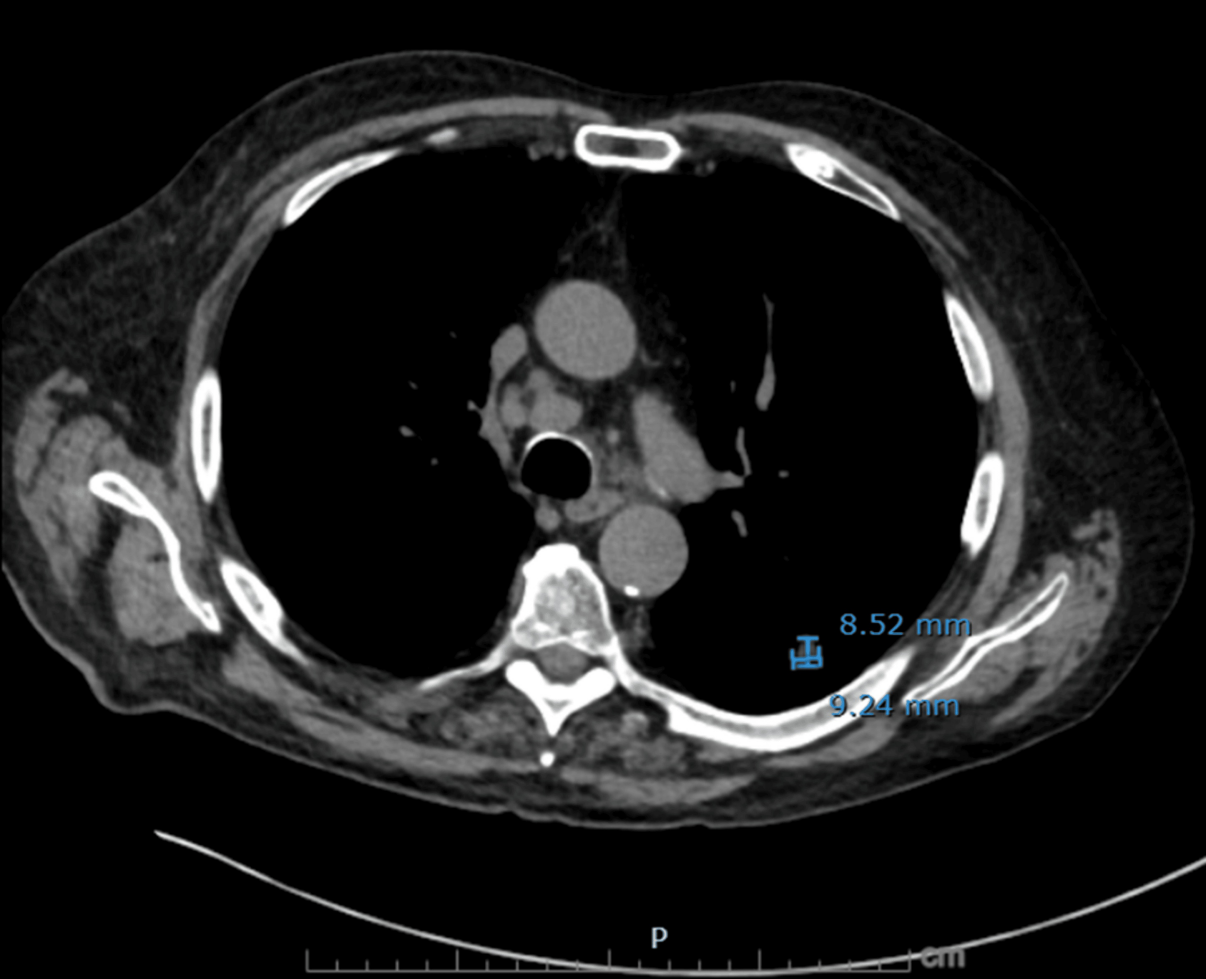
Axial non-contrast CT chest demonstrating residual treated left lower lobe lesion A spiculated nodule in the left lower lobe measuring 9.2 mm (AP) × 8.5 mm (transverse) on image 60, slightly decreased from the prior measurement of 9.3 × 10.6 mm, corresponding to the site of previously treated squamous cell carcinoma.
